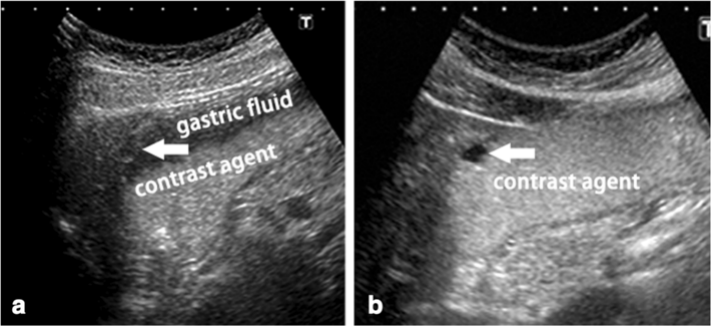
As compared with water, the uniformity of the mid to high echoic contrast agent may improve ultrasound visualization of hypoechoic gastric tumors. a Because the echo intensity of the tumor was similar to that of the gastric fluid floating above the cellulose-based contrast agent, visualization of the hypoechoic tumor was blurred and unclear. b The uniformity of the mid to high echoic contrast agent could improve ultrasound visualization of the hypoechoic gastric tumors. Arrow, pointing at the hypoechoic tumor
